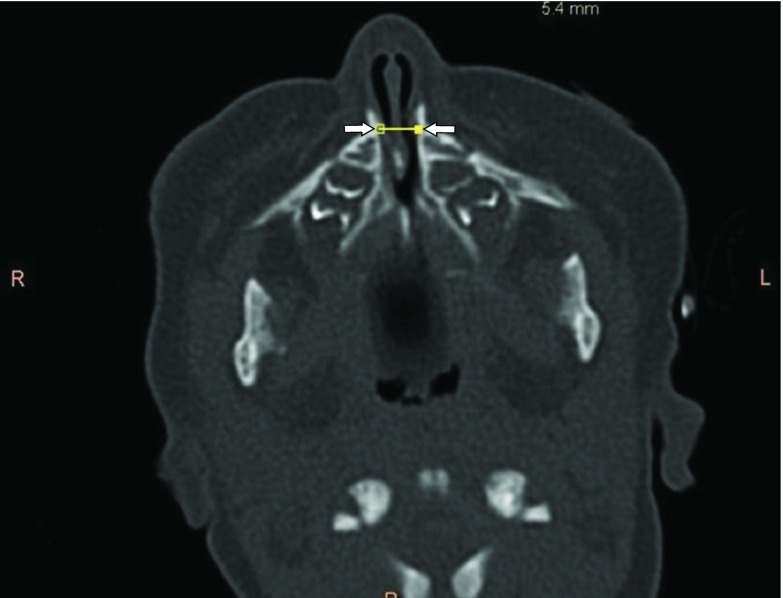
CT image (pre-stenting): axial view of the narrowed pyriform aperture measuring 5.4 mm. Note the medially angled maxillary spines (white arrows).
